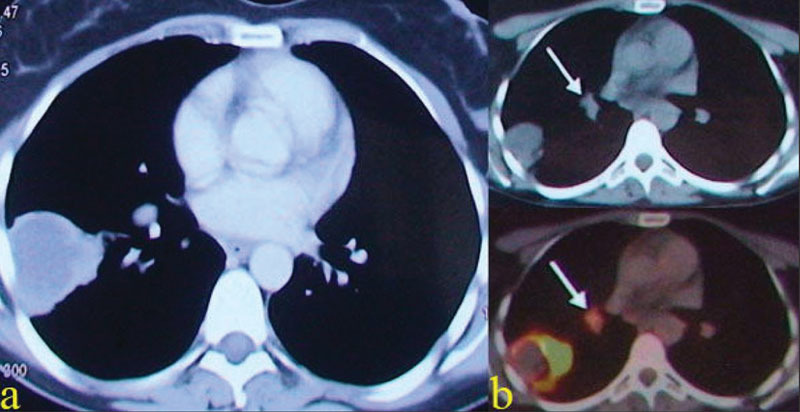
(a) Thorax computed tomography (CT) after antibiotic therapy and (b) positron emission tomography/CT after antibiotic and antituberculosis therapy showing the mass and hilar (no. 10) lymph node (white arrow).
